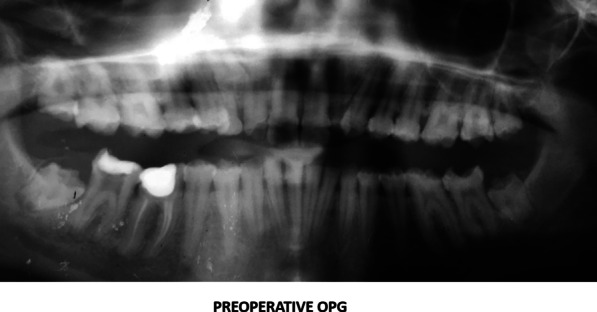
Preoperative orthopantomogram view showing root canal treated tooth with respect to 36 and the endodontic treatments to be performed on #37, 47,47,16,26
